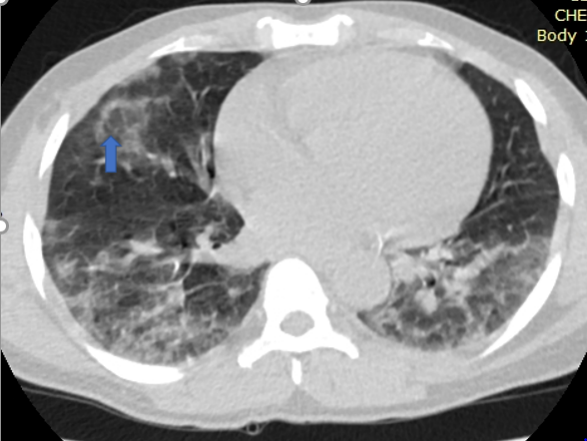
An axial non-enhanced chest CT scan of a 52-year-old man with breathlessness and cough of 14-days duration. Lung window image shows a reversed halo sign (arrow) with bilateral posterior basal consolidation. RT-PCR test confirmed COVID-19.
